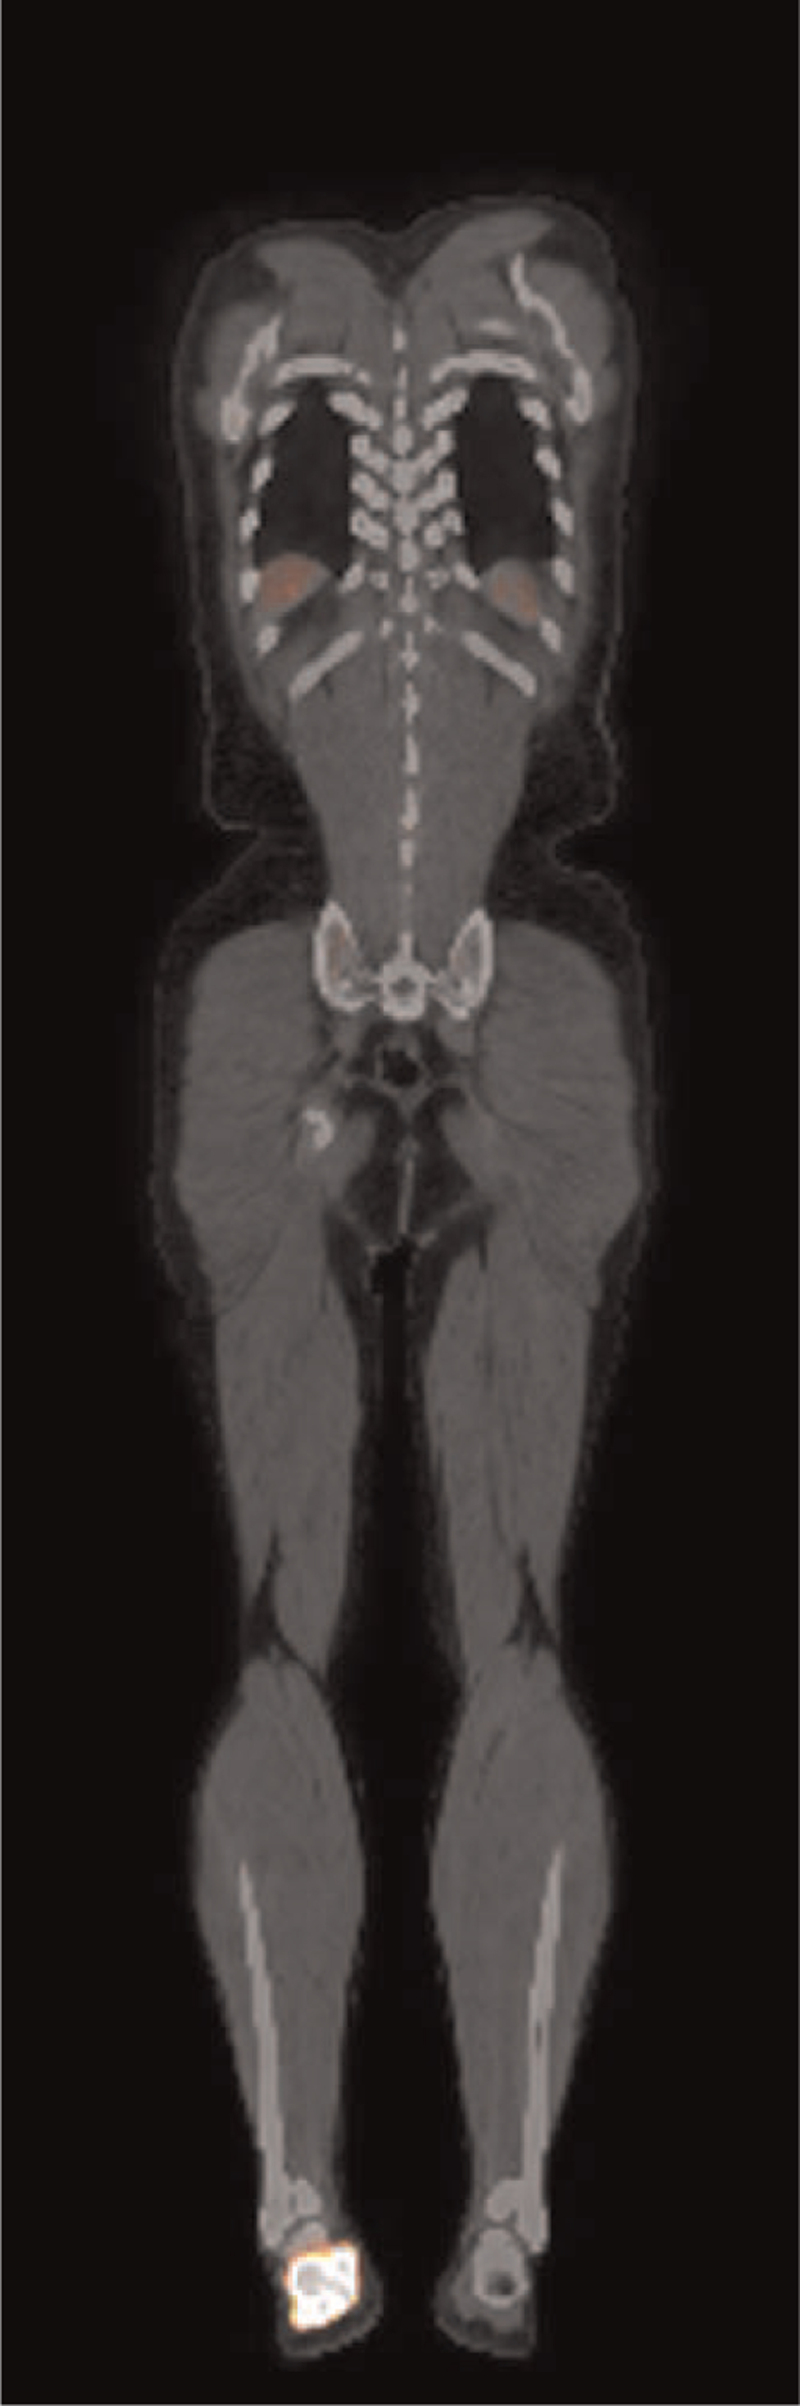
An anterior view of 18F-FDG PET images reveals an increased uptake in the calcaneus (SUVmas 6.6). No metastasis was found. PET = positron emission tomography.
